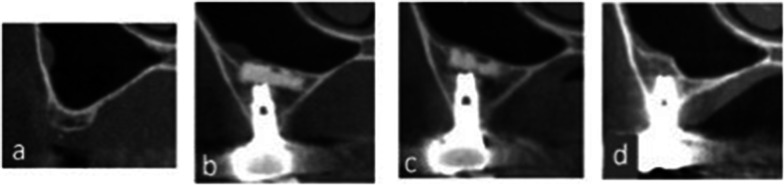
Representative frontal X-ray images of patient no. 3. a Before surgery. b 1 year after surgery. c 2 years after surgery. d 5 years after surgery
